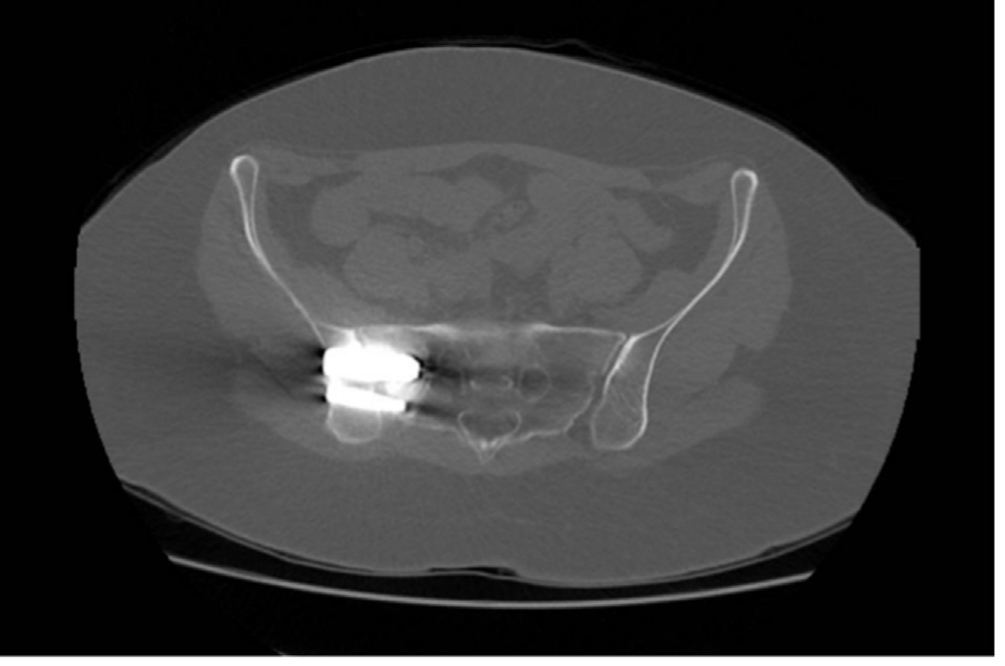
Axial cuts of a CT scan of pelvis, showing sacral osteolysis around caudal implants on the left side.
